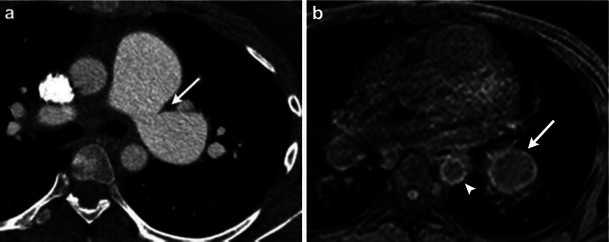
Contrast-enhanced axial CT image (a) in a 16-year-old patient with progressive dyspnea and absent left upper extremity pulse shows a focus of smooth narrowing and aneurysmal dilatation of the left main pulmonary artery (*arrow*). Late venous phase axial MR image from a 3D GRE acquisition (b) shows delayed enhancement of an aneurysmal left pulmonary artery branch (*arrow*). Also note the wall enhancement of descending thoracic aorta (*arrowhead*) consistent with vasculitis. These findings are suggestive of Takayasu arteritis
